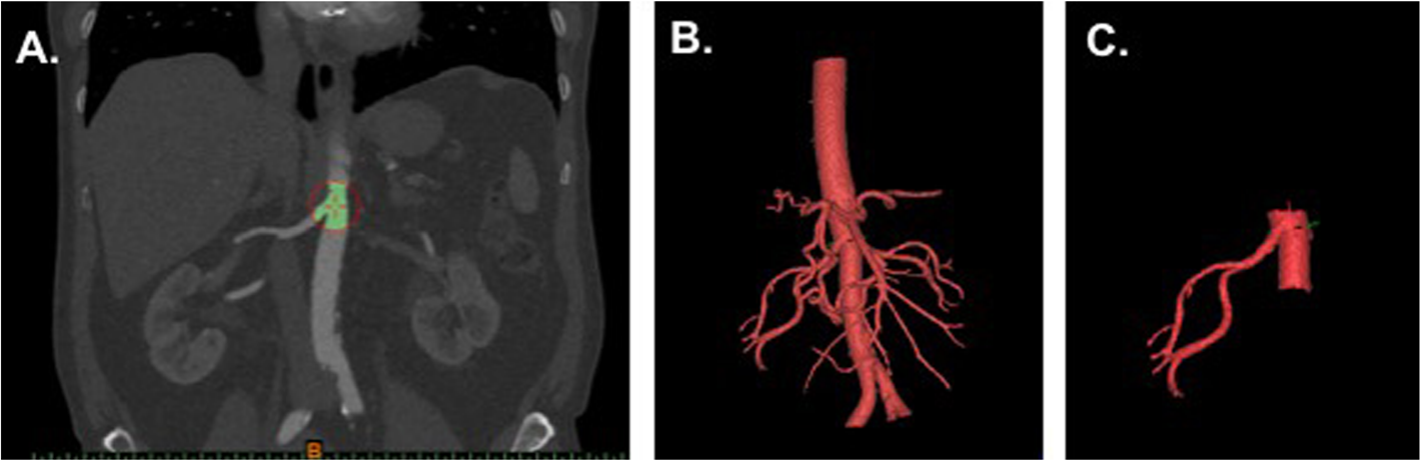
a Coronal CT image showing aorta and right renal artery selection. b 3D visualization of segmented arterial structures. c Remaining arterial region after trimming has been performed
